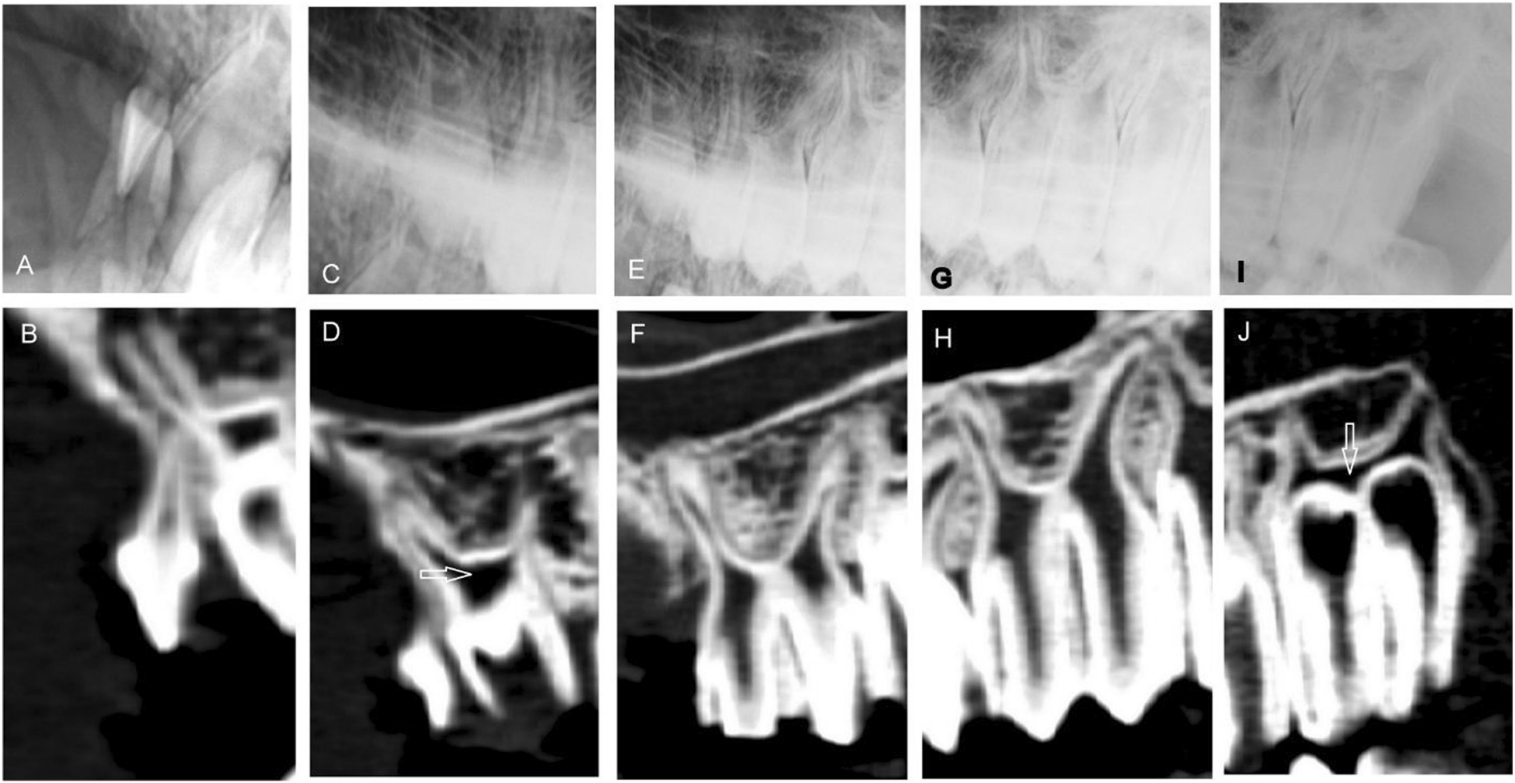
Maxillary cheek teeth. Maxillary cheek teeth of an adult llama (age 44 months). There were two premolars and three molars on each side. A/C/E/G/I: Left to right lateral30°dorsal - lateroventral oblique projection showing the right maxilla. B/D/F/H/J: Sagittal CT reconstructions. A and B: 07, C and D: 08, E and F: 09, G and H: 10, I and J: 11. Image D and J show a clear and obvious common pulp chamber (white arrow).
